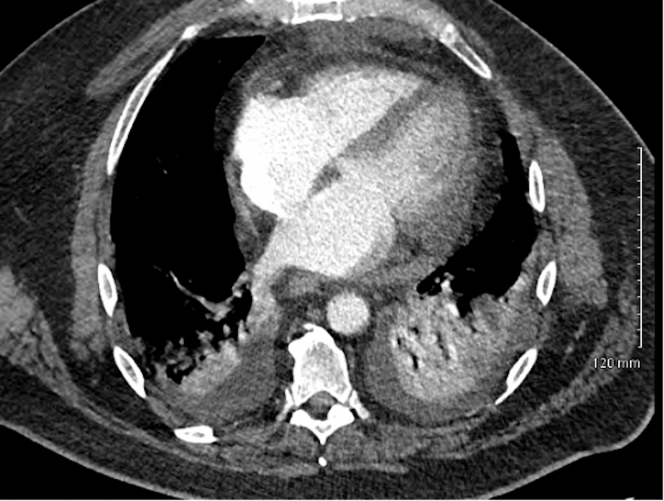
Chest Computed Tomography Angiography Demonstrating Right Ventricular Enlargement Axial contrast-enhanced imaging shows a right ventricular–to–left ventricular diameter ratio of 1.0 with straightening of the interventricular septum.
